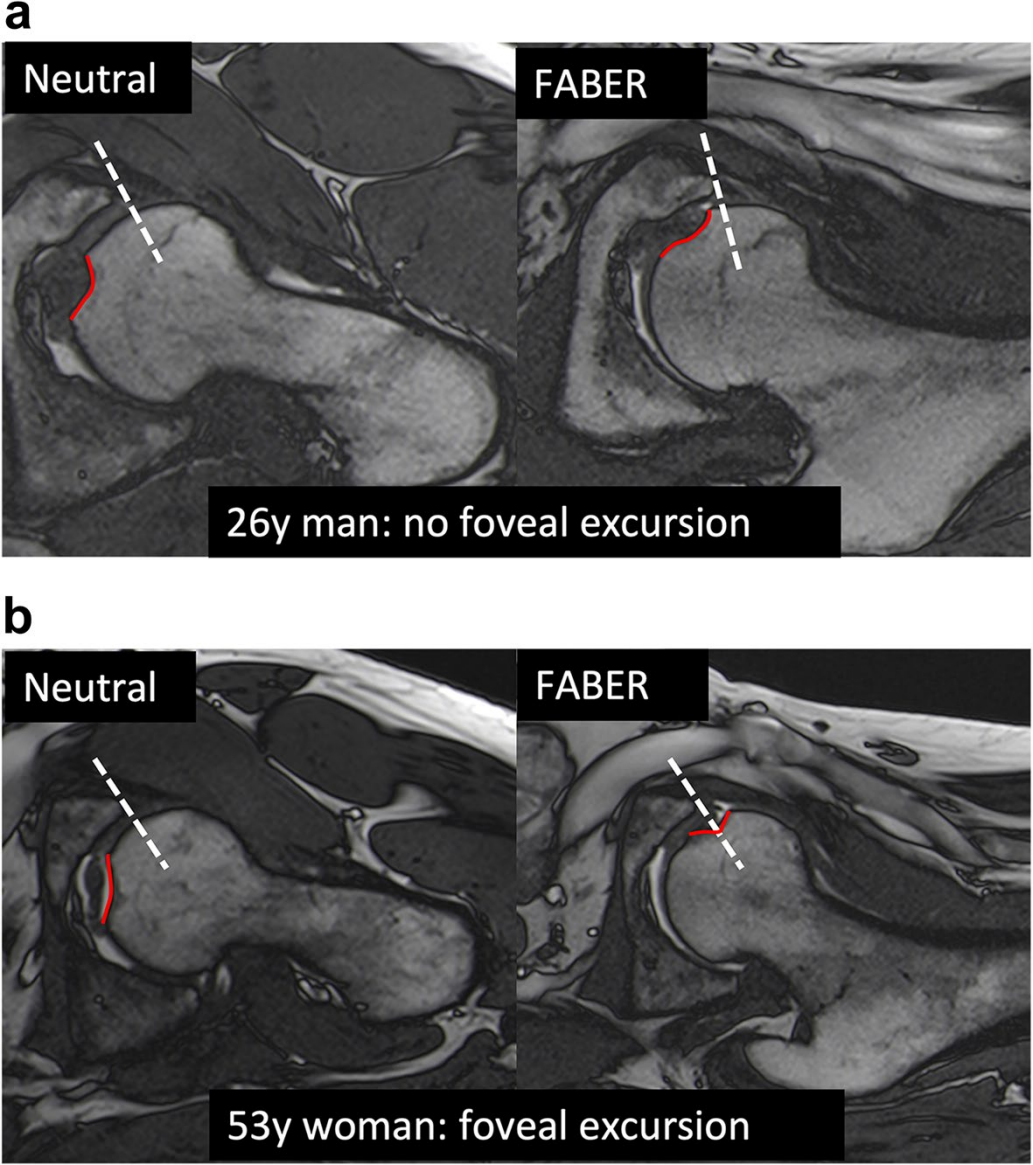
Assessment of foveal excursion on axial-oblique T2-weighted True FISP images. a No foveal excursion: In both neutral (left) and FABER (right) positions, the fovea capitis femoris (solid red line) is not crossed by a line perpendicular to the anterior acetabular rim (dashed white line). b Foveal excursion: The fovea capitis femoris (solid red line) is crossed by the line perpendicular to the anterior acetabular rim (dashed white line) in the FABER position
